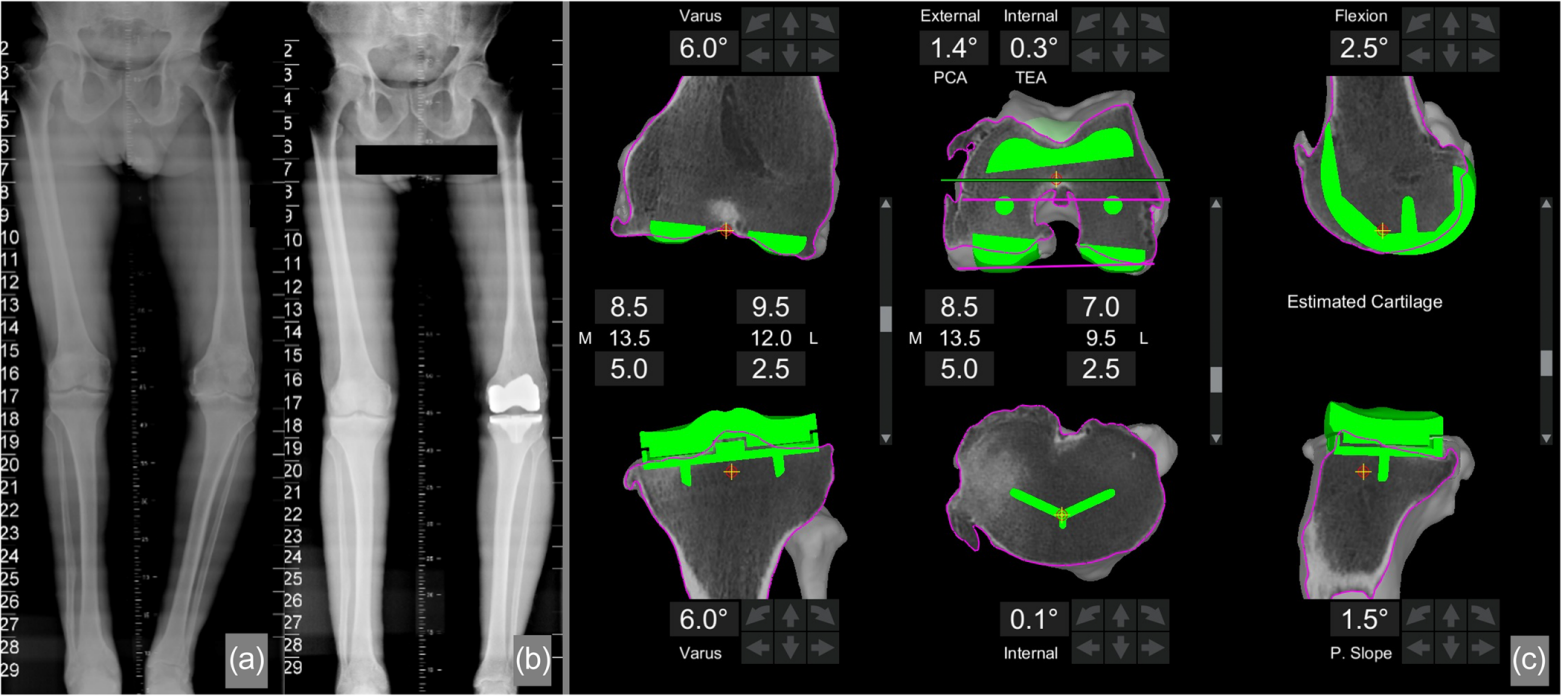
Seventy‐six‐year‐old female patient with a major varus coronal deformity, presenting a preoperative mechanical hip–knee–ankle (mHKA) angle of 161° on the left side, corrected to 167° after total knee arthroplasty (TKA). (a) Preoperative long‐leg standing radiograph; (b) postoperative long‐leg standing radiograph; (c) intraoperative screenshot of TKA performed with the Mako® robotic system (Stryker ®), showing planning adjustments according to functional knee positioning principles.
